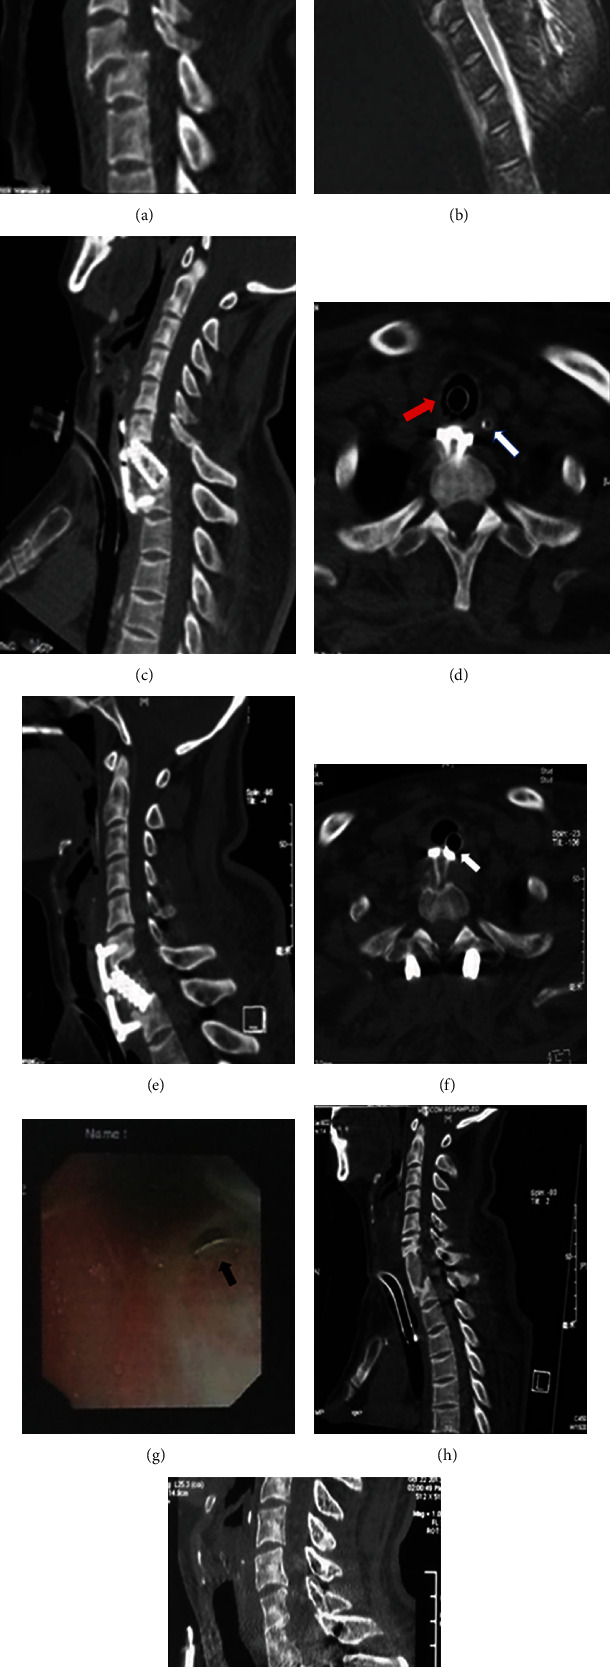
Images of case 1. (a) Preoperative sagittal CT reconstruction showing the dislocation of C6 and fracture of C7; (b) T2-weighted sagittal MR showing the disruption of the discoligamentous elements from the anterior longitudinal ligament to the nuchal ligament and spinal cord injury (as shown by the extensive high signal intensity of the spinal cord); (c) postoperative sagittal CT reconstruction revealing the dislocation of the C6 and the failure of the instrumentation; (d) postoperative axial CT scan showing the back out of the screws and the encroaching of the plate on the trachea. The white arrow indicates the esophagus, with the gastric tube inside, and the red arrow indicates the trachea, with the endotracheal tube inside; (e) after posterior revision, sagittal CT reconstruction was performed, showing the restoration of the cervical alignment, but the anterior implant failure was more significant; (f) axial CT scan after posterior revision showing the encroachment of the plate on the trachea, with the plate in contact with the endotracheal intubation (white arrow); (g) flexible bronchoscopy after posterior revision showing the perforation of the posterior tracheal wall with the screws/plate penetrating into the lumen of the trachea (black arrow); (h) sagittal CT reconstruction after the anterior revision showing the strut bone graft between C6 and T1 and a more distal tracheostomy tube; and (i) sagittal CT reconstruction showing the maintained alignment of the cervicothoracic junction and the solid fusion between C6 and T1 16 months after the anterior revision procedure.
